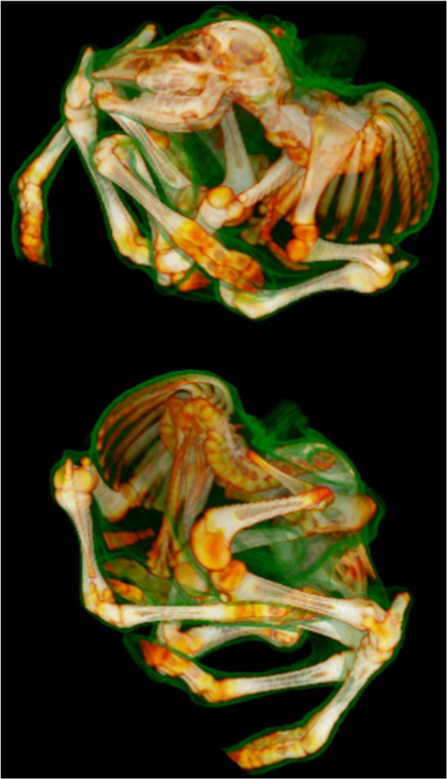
Surface rendered computed tomography images in two projections of a case of arthrogryposis multiplex congenital (AMC). The scanning data are rendered with bone and soft tissue with the latter set to have a degree of transparency in the reconstruction thus allowing visualisation of the calf’s overall morphology and its relation to the underlying skeletal abnormalities. Images prepared using OsiriX: An open-source software for navigating in multidimensional DICOM images
